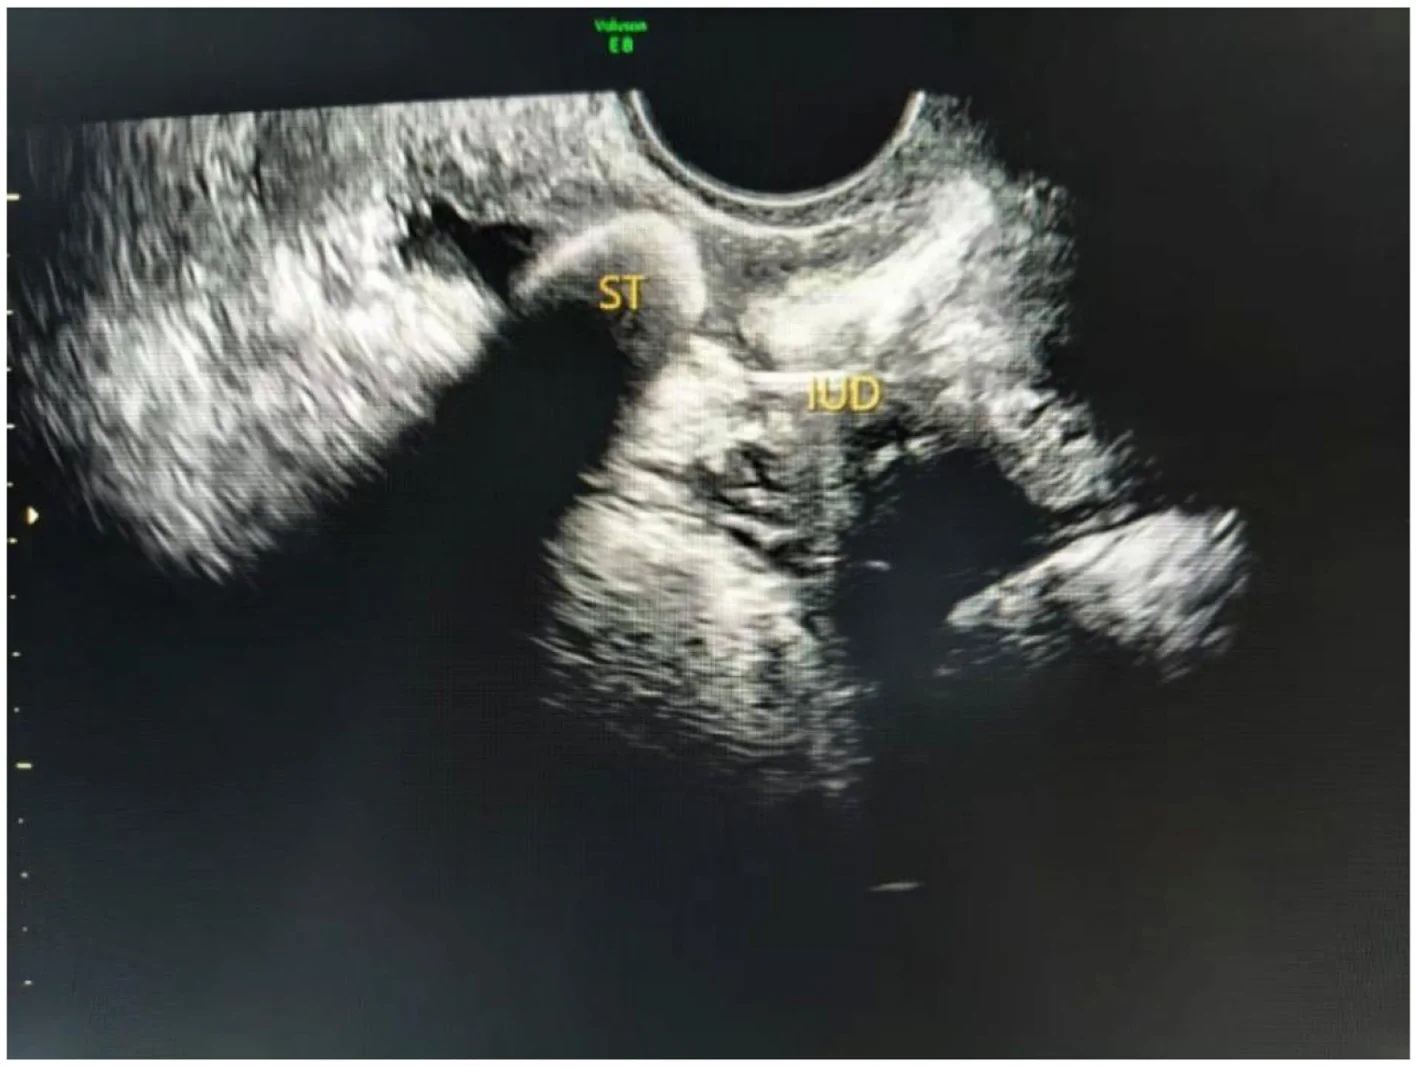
Transvaginal ultrasound image demonstrating bladder stone formation secondary to IUD migration. Sagittal view showing multiple intensely echogenic foci with prominent posterior acoustic shadowing (asterisks) within the bladder lumen. The stones are fixed in position at the site of IUD penetration and do not shift with changes in patient positioning or transducer pressure. Transverse view demonstrating localized bladder wall thickening with heterogeneous echogenicity at the stone attachment site, consistent with chronic inflammatory and fibrotic changes.
